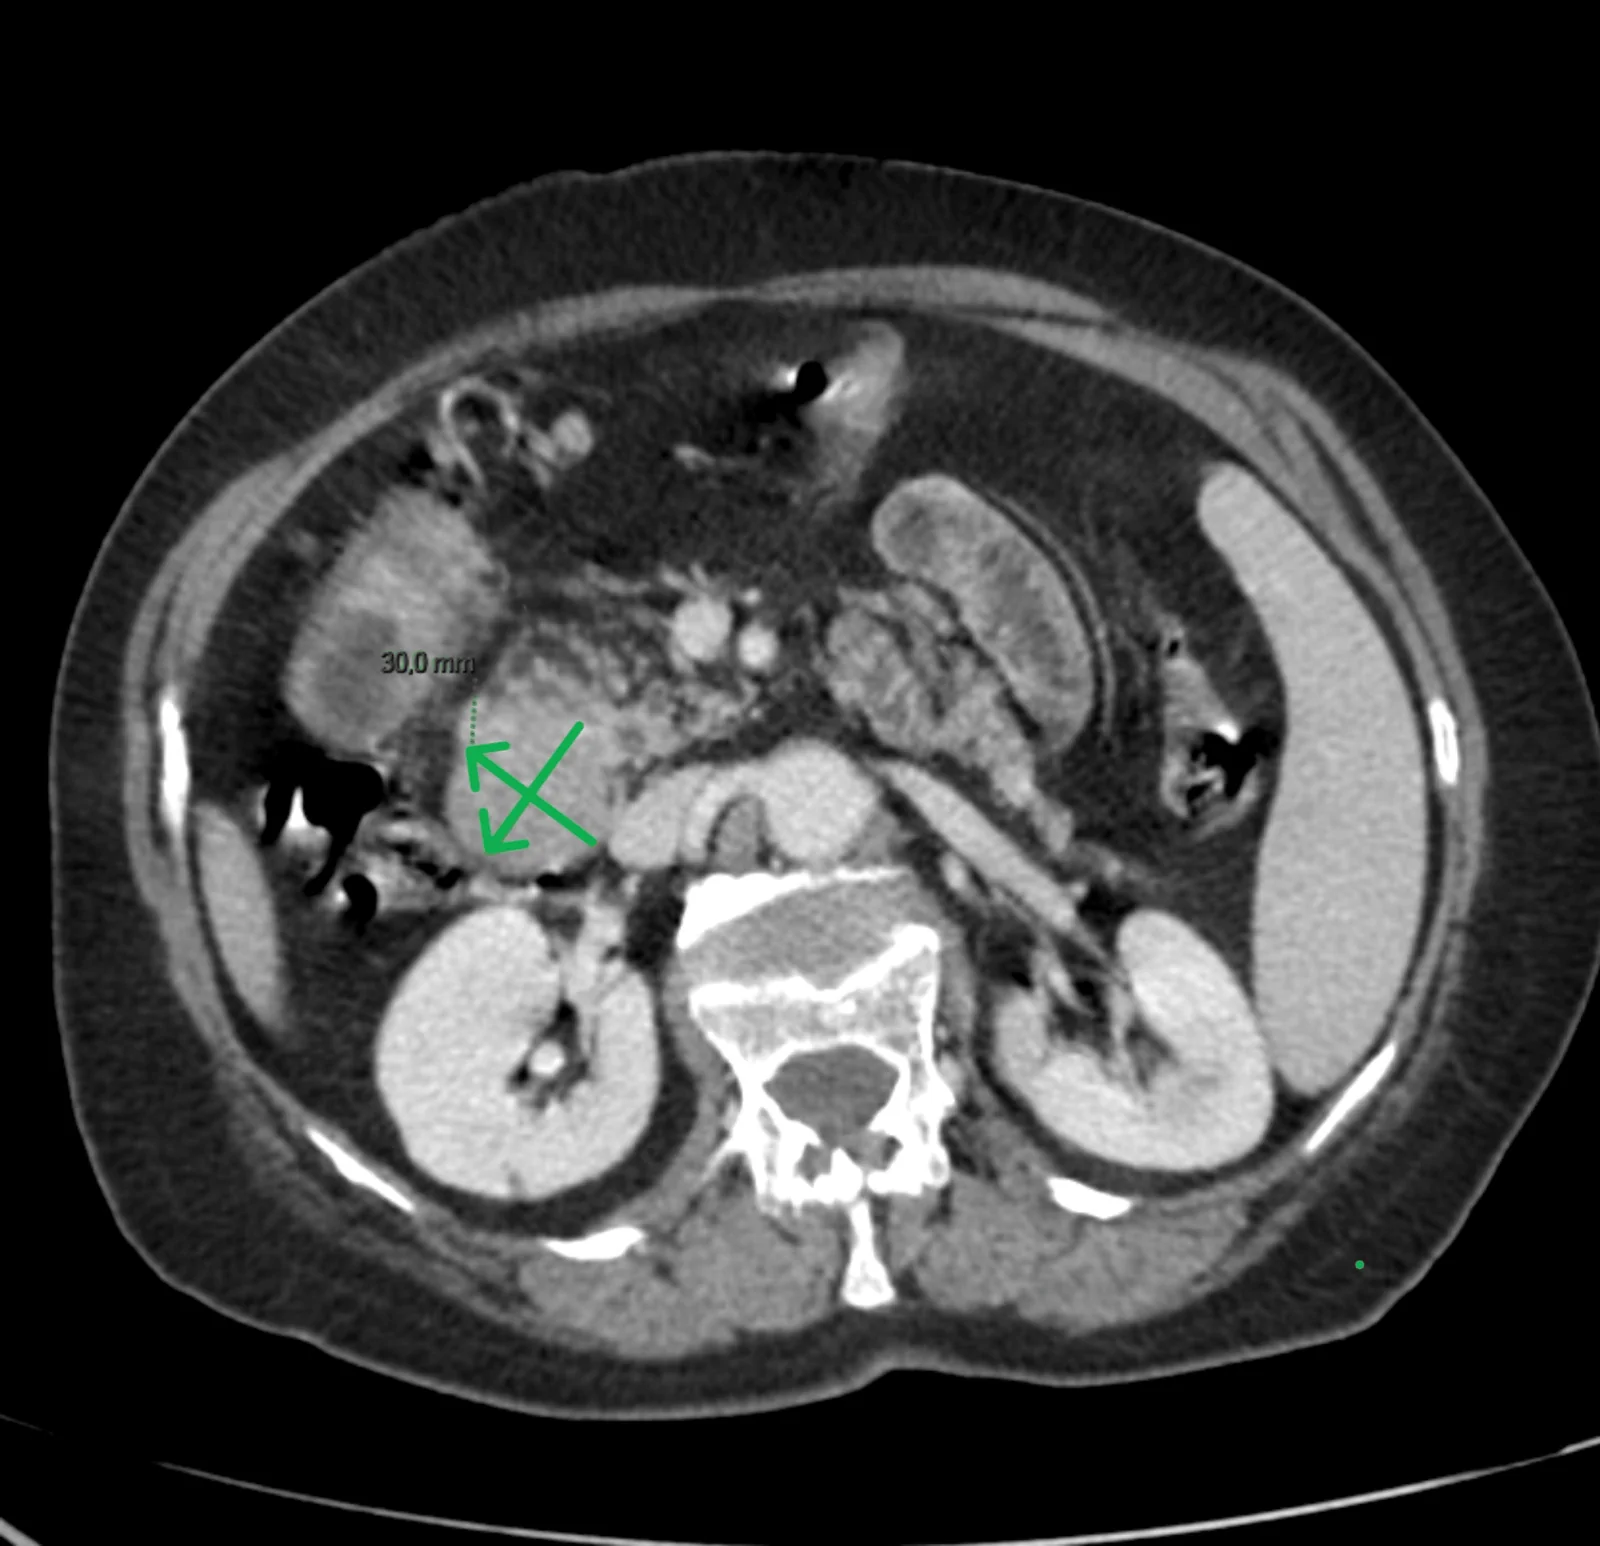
Contrast-enhanced abdominal computed tomography, axial view, showing an endoluminal polypoid soft-tissue mass measuring 30 mm (green arrows).
